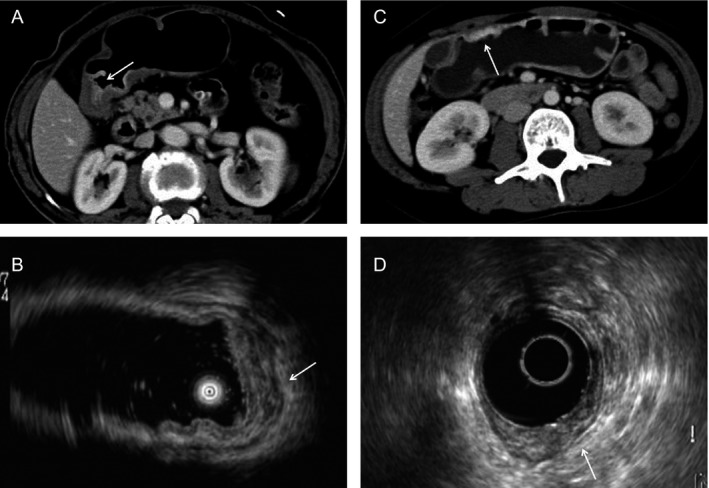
Contrast‐enhanced computed tomography images and corresponding endoscopic ultrasonography (EUS) images of early gastric carcinoma. (A, B) 72‐year‐old woman with early SRC. Contrast‐enhanced CT scan obtained during the parenchymal phase shows focal inner wall thickening (arrow). EUS image of the lesion shows an echo‐poor, inhomogeneous lesion. Surgical resection confirmed signet ring cell carcinoma infiltrated to the mucous layer. (C, D) Elevated early NSRC in a 38‐year‐old woman. The attenuation of the enhancing thickened gastric wall is higher than that of the SRC (arrow). EUS image of the lesion shows a hypoechoic lesion spreading from the mucosal to submucous layers.
